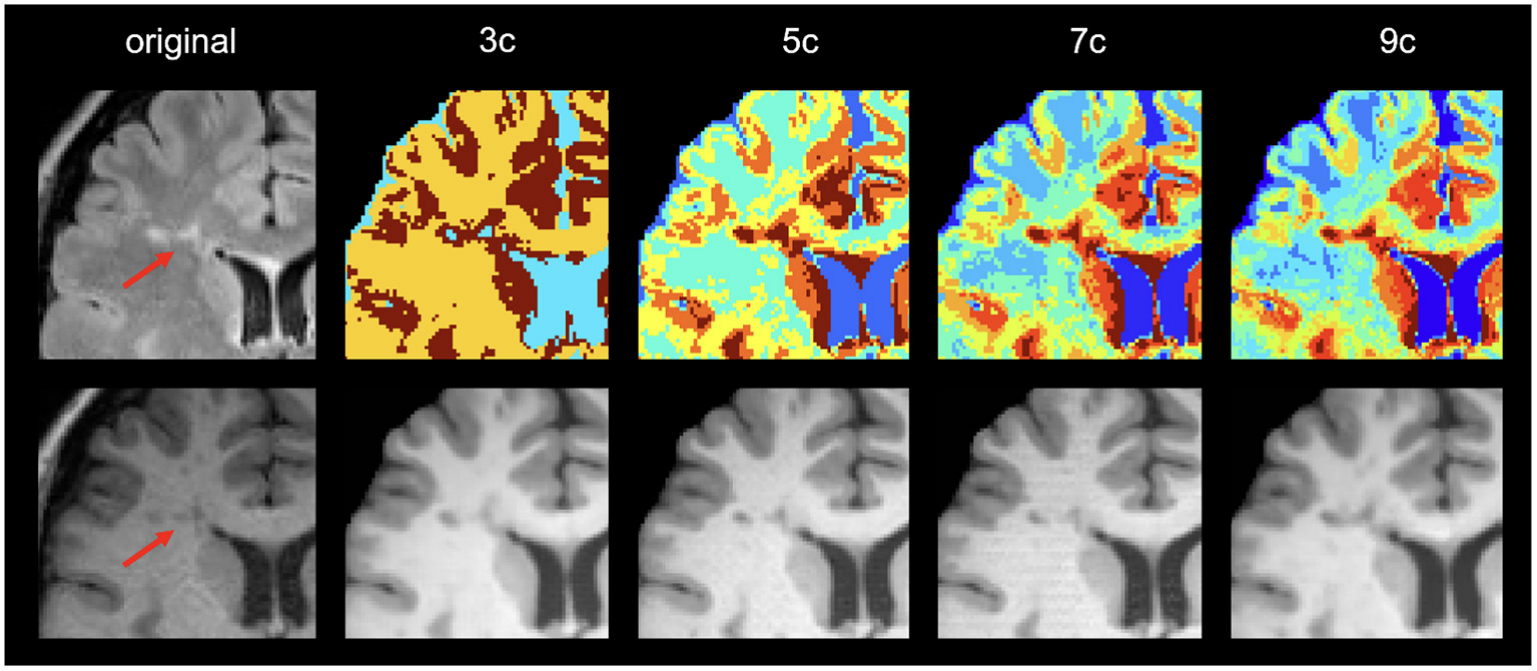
Example of image generations in a lesion area. First column shows the original T2-FLAIR and T1 image. The rest of the columns show the clustering result and the corresponding generated image using different numbers of clusters (3, 5, 7, and 9, respectively).
